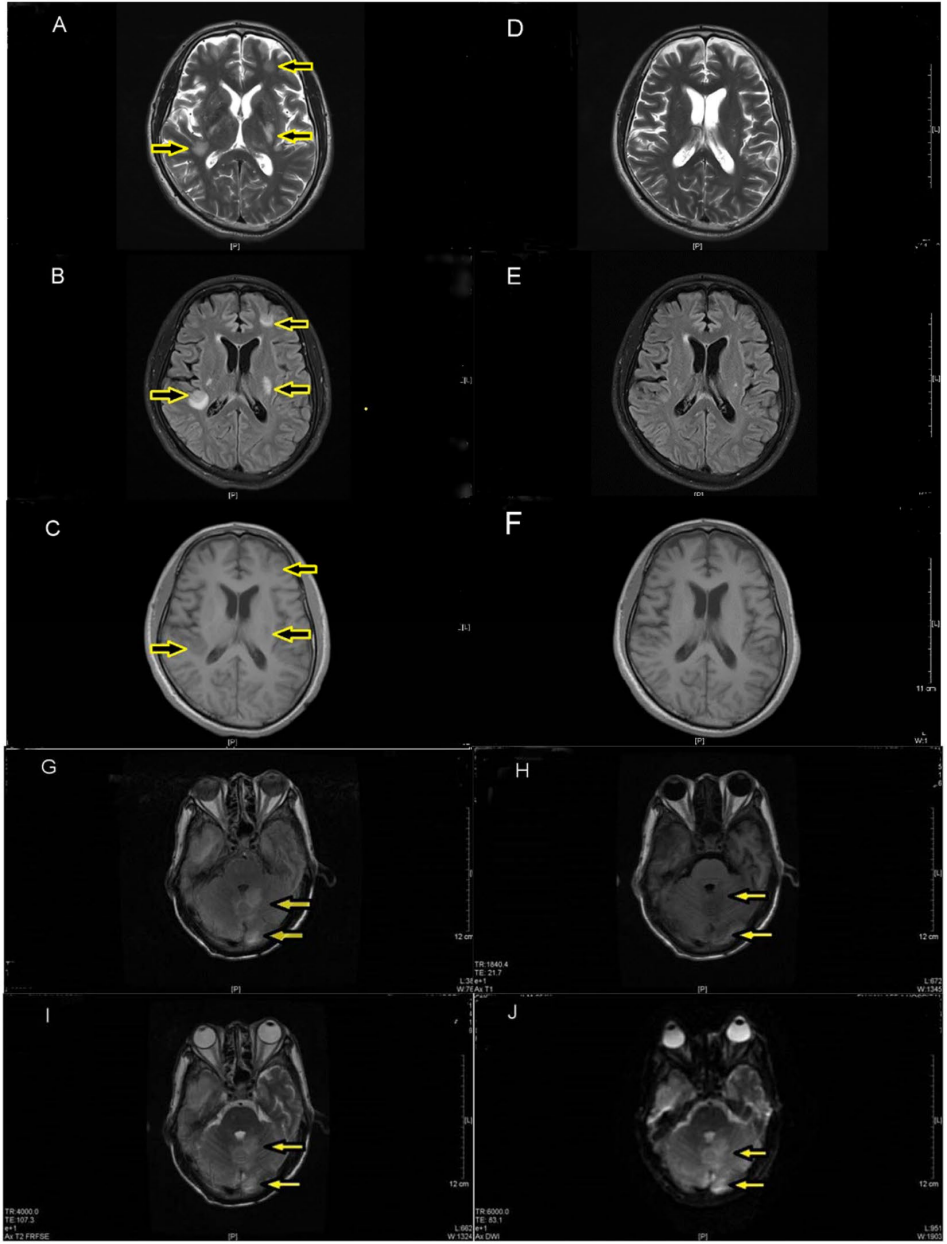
Supratentorial lesions as showed in picture A, B, C, D; Picture A, B, C are T1W1 imaging, T2W1 and Flair imaging respectively of a patient diagnosed with CM before being treated with GCs, 43th days after being admitted to hospital. T1W1 displayed low signal while T2W2 and Flair imaging displayed high signal which distributed beneath the left frontal cortex, within the white matter of basal ganglia and the right cistern (as indicated by arrows). Picture D, E, F are the same T1W1 imaging, T2W2 and Flair imaging of the patient after being treated with GCs for 21 days. No significant abnormality was seen in T1W1 and T2W2 imaging while the high signal in the left frontal cortex and the white matter of right cistern showed by Flair was obviously decreased or even disappeared in comparison to the previous (relatively compare with the area indicated by arrows in Picture A, B, C). Infratentorial lesions showed in picture G, H, I, J by Flair, T1W1, T1W2 and DWI imaging, the yellow arrow represents inflammatory signal. In this figure they weren’t the same person for pictures of supratentorial and infratentorial lesions.
